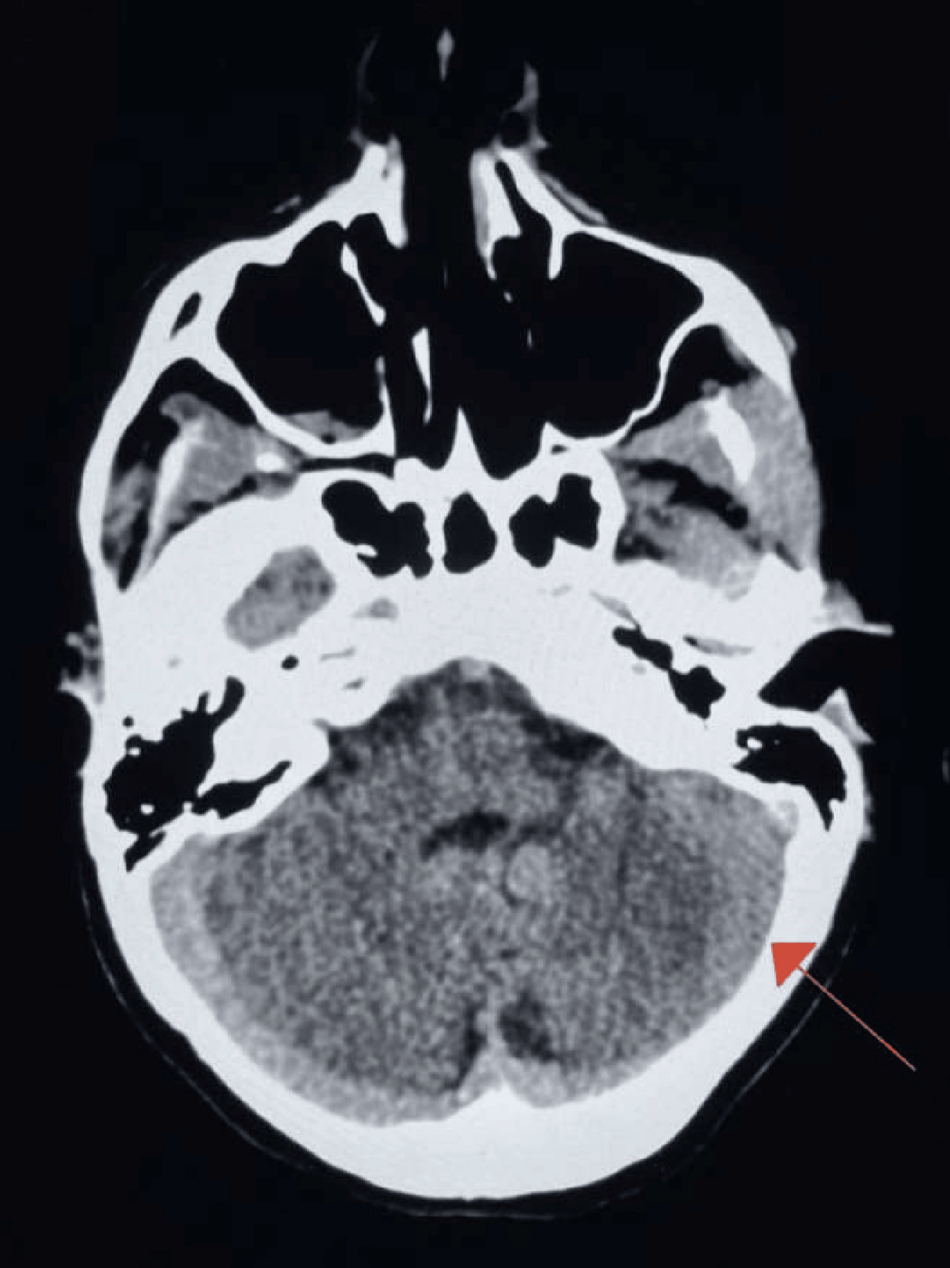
CT imaging of the head in the axial plane showing hypoattenuation of the left sigmoid sinus (red arrow). Before confirming the diagnosis of MELAS syndrome, a CT venogram was recommended for a more sensitive evaluation to rule out venous sinus thrombosis.
